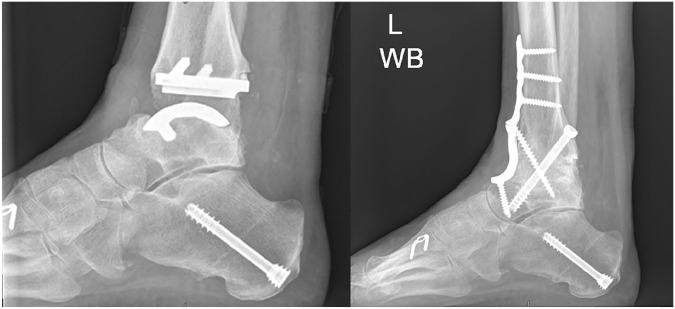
Pre- and postoperative lateral radiographs demonstrating tibiotalar fusion using an anterior ankle approach + screws. Preoperatively there was collapse of the talar dome with cystic change in both the tibia and talus. Radiographs confirm solid graft incorporation with no loss of height.
